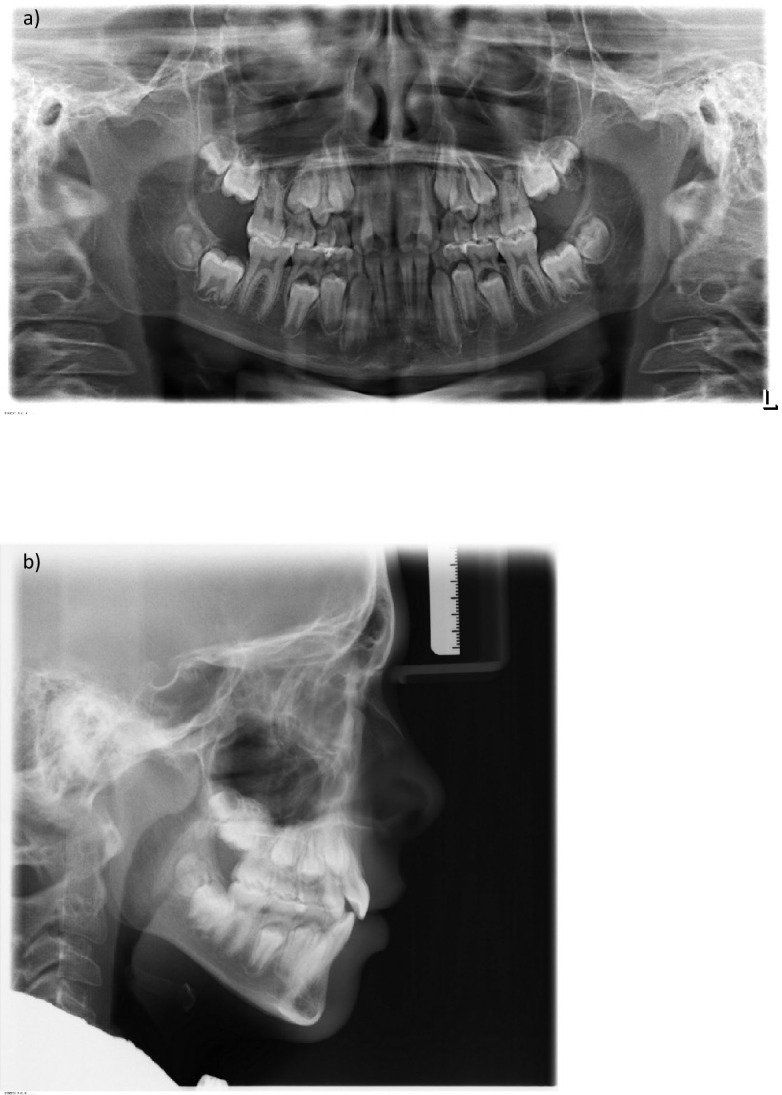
a Panoramic X-ray at the start of treatment b lateral cephalogram at the start of treatment
